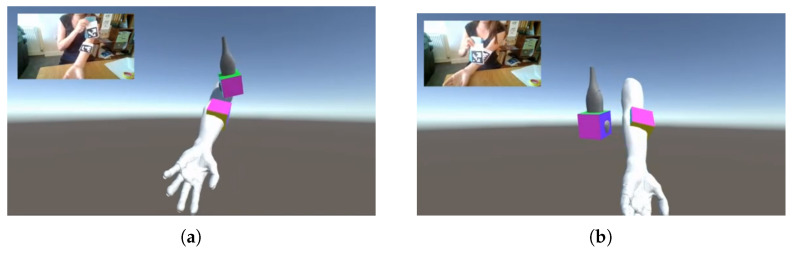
Issue of occlusion when using two ArUco markers for the tracking. (a) Tracking of the arm and the ultrasound probe using ArUco markers; no occlusion. (b) Tracking of the arm and the ultrasound probe using ArUco markers; occlusion of the arm marker by the probe marker and impact on the tracking of the arm in the virtual scene which no longer follows the arm of the student.
